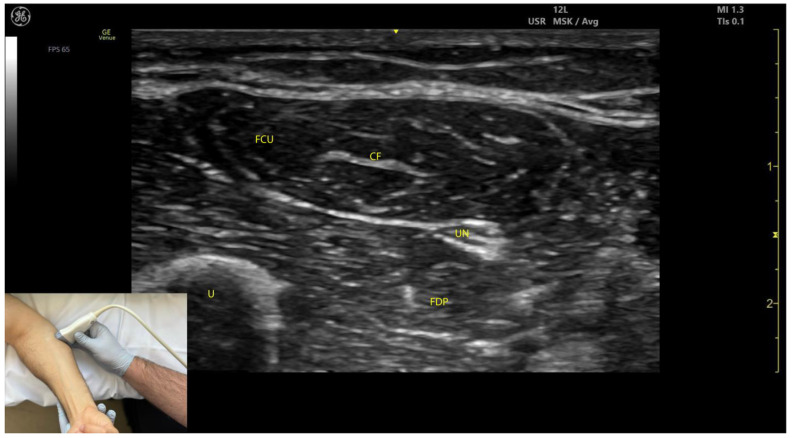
Ultrasound anatomy of the flexor carpi ulnaris (FCU) with key landmarks: FCU—flexor carpi ulnaris; UN—ulnar nerve; FDP—flexor digitorum profundus; U—ulna; CF—cloud fascia.
